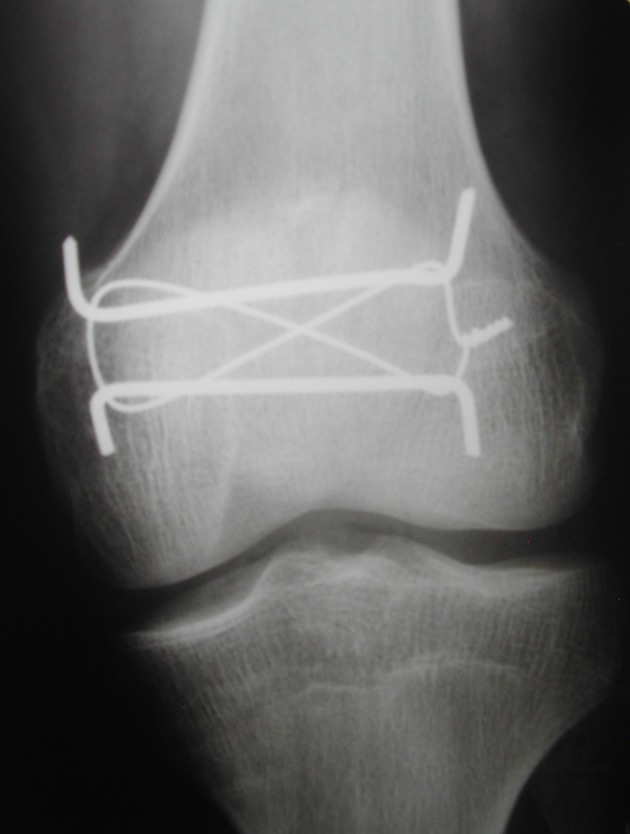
Seven days postoperatively, anteroposterior radiograph demonstrated the reduction of the separated fragments and the 3 mm distance between the fragments was gone. This incidence has shown better modified tension band.
